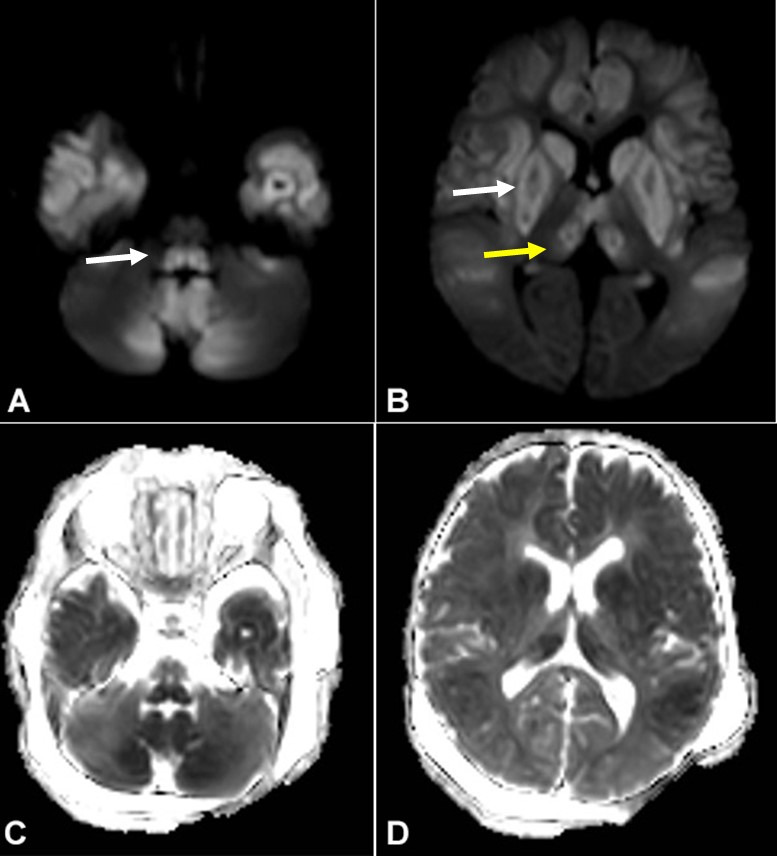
Axial diffusion-weighted imaging (DWI) (A and B. b = 1000 s/mm^2^) and apparent diffusion coefficient (ADC) maps (C and D) show bilateral diffusion restriction involving brainstem dorsal tracts (white arrow, A), basal ganglia (white arrow, B), medial thalami (yellow arrow, B), cerebellum and diffuse cerebral gyral swelling (more marked in fronto-temporal regions).
